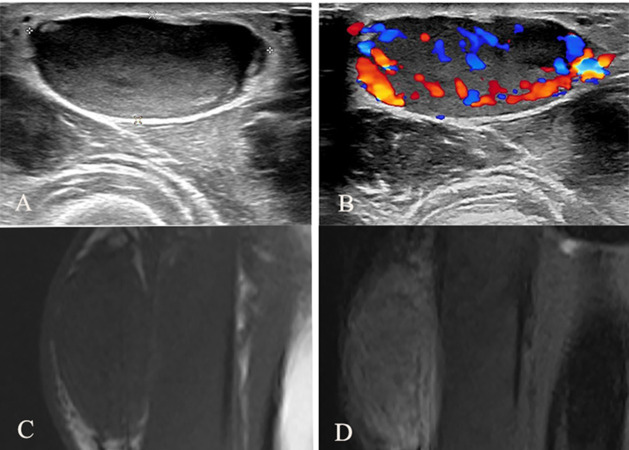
Ultrasound doppler and MRI of the mass: (A) 2D ultrasound shows a hypoechoic mass measuring approximately 34×15mm in the right forearm subcutaneous tissue, with well-defined borders and heterogeneous internal echogenicity. (B) CDFI reveals abundant blood flow signals. (C) T1WI weighted image demonstrates an oval-shaped abnormal signal with clear margins, measuring 1.7×3.7×3.2cm in size, showing relatively homogeneous internal signals with slightly hypointense characteristics. (D) T2WI fat-suppressed sequence displays slightly hyperintense signals.
